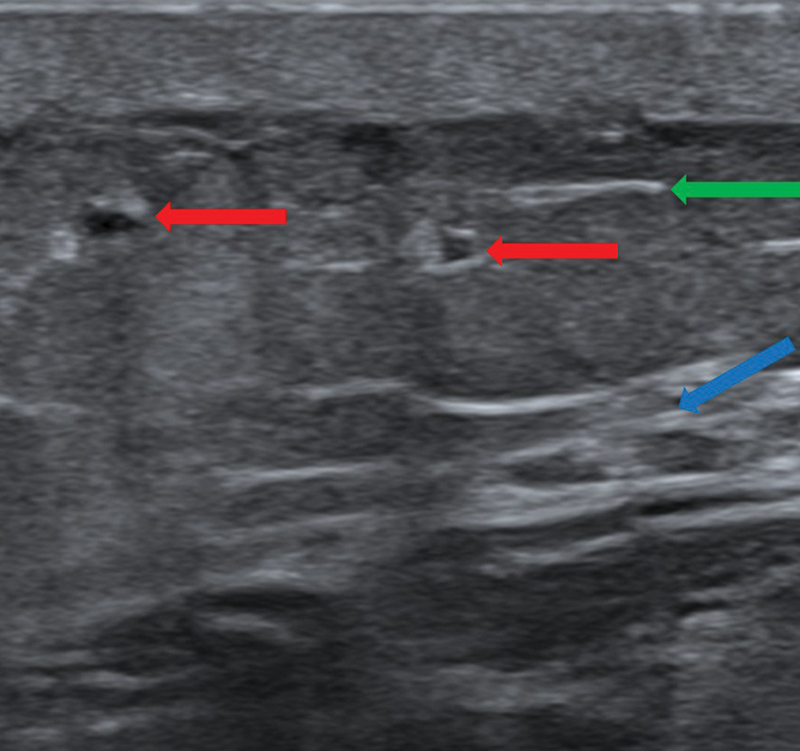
Ultrasonography of lymphatic vessels. A 22 MHz hockey stick probe was used. Red arrows show the lymphatic vessels. The green arrow shows the superficial fascia. The blue arrow shows the deep fascia.
